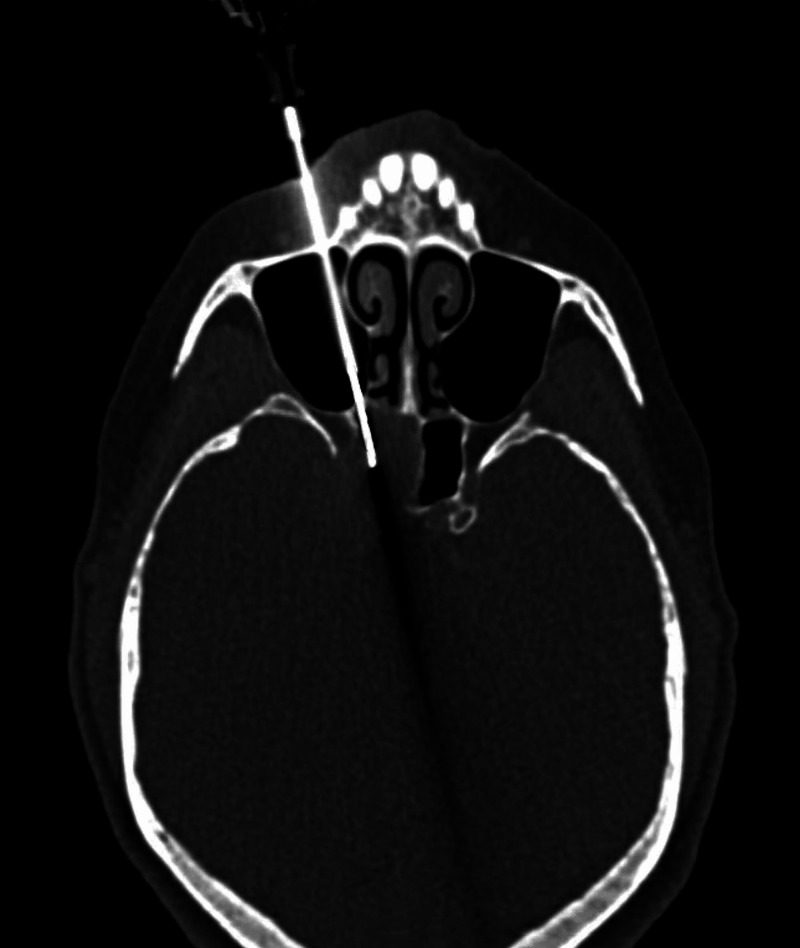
Axial, intraoperative, brain CT showing the path of the guiding needle through the right maxillary sinus and the right sphenoid sinus to the right cavernous sinus plasmacytoma. CT, computed tomography
